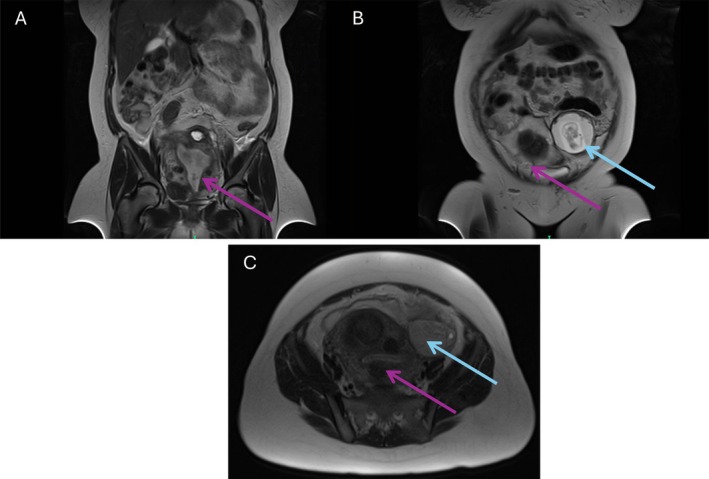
MRI images. (A, B) Coronal view of multi‐fibroid uterus (pink arrow) and pregnancy sac (blue arrow). (C) Axial view of uterus with left sided gestation sac visible. MRI showing the left adnexal pregnancy. The left ovary was seen separately. There was peripheral haemorrhage surrounding the gestation sac with a moderate intraperitoneal haematoma and free fluid within the perihepatic space and paracolic gutters.
